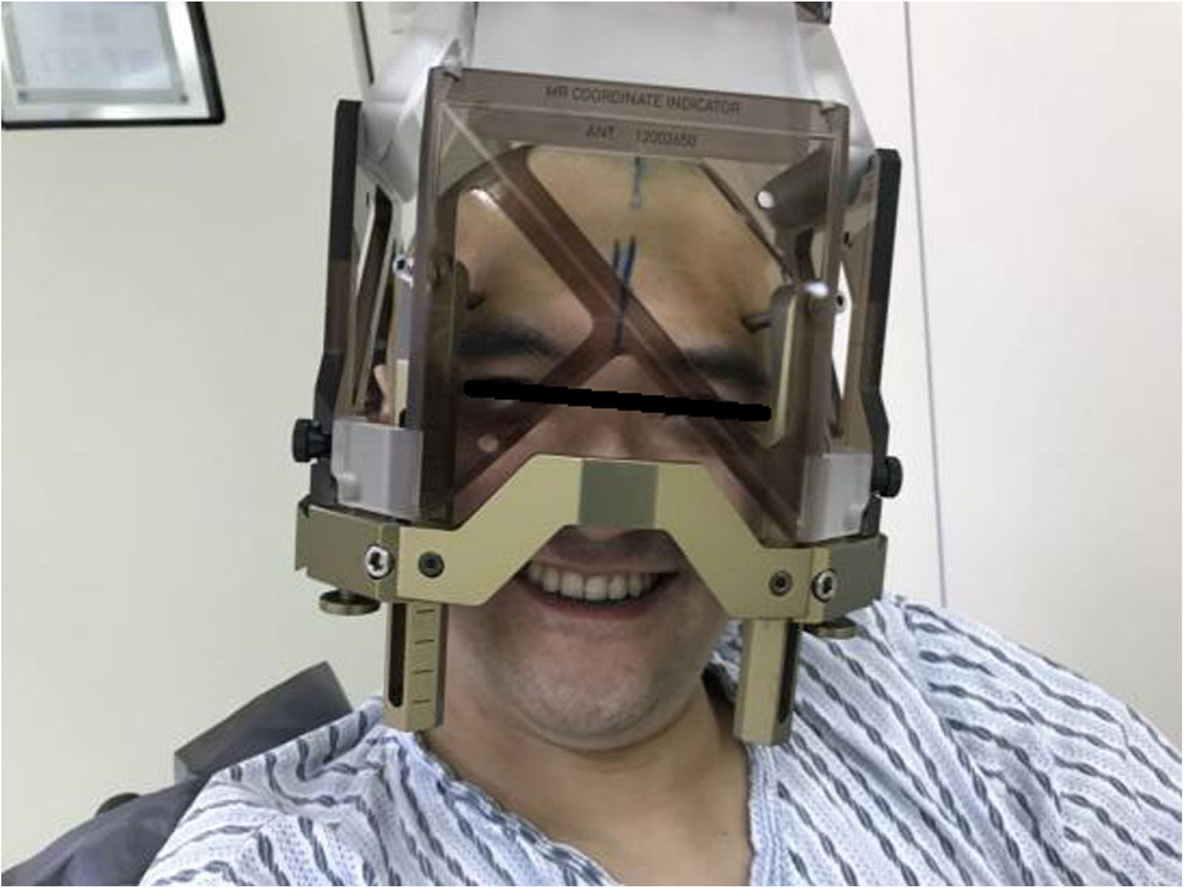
MRI was performed to locate the lesions after preoperative installation of the leksell headgear
